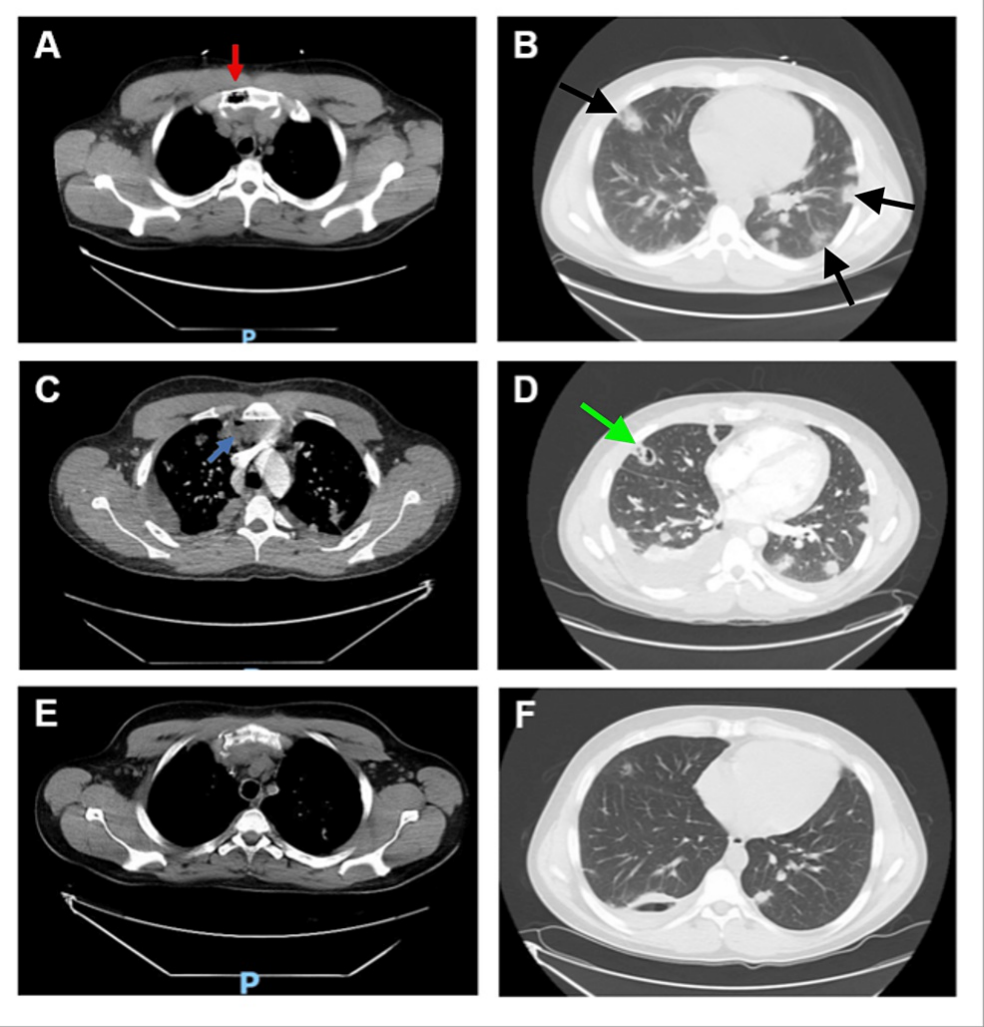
Computed tomography (CT) scans of the chest on day Four (A, default window; B, lung window), day 11 (C, default window; D, lung window), and day 53 (E, default window; F, lung window). On day four, the CT chest demonstrated intraosseous gas and edema in the right aspect of the manubrium of the sternum (red arrow), consistent with emphysematous osteomyelitis (A). Widespread nodules were visible in the bilateral lung fields, consistent with septic emboli (black arrows) (B). On day 11, CT chest demonstrated new mediastinal abscesses in front of and behind the sternum (blue arrow) (C) and new right and left pleural effusions (C, D). Some pulmonary nodules had evolved into cavitary lesions (green arrow) (D). On day 53, the air within the manubrium had resolved (E), and marked improvement in the lung lesions was observed (F).
